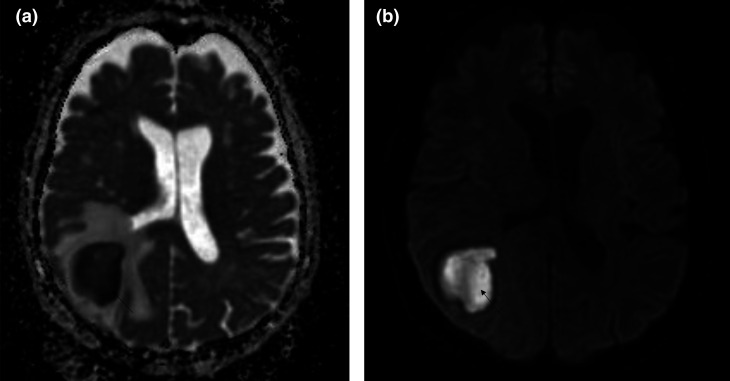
Restriction of diffusion at the mass level and lack of restriction at the capsule level argues for an infectious process. (a): Apparent Diffusion Coefficent (ADC) sequence (b): Diffusion-weighted imaging (DWI) sequence.
